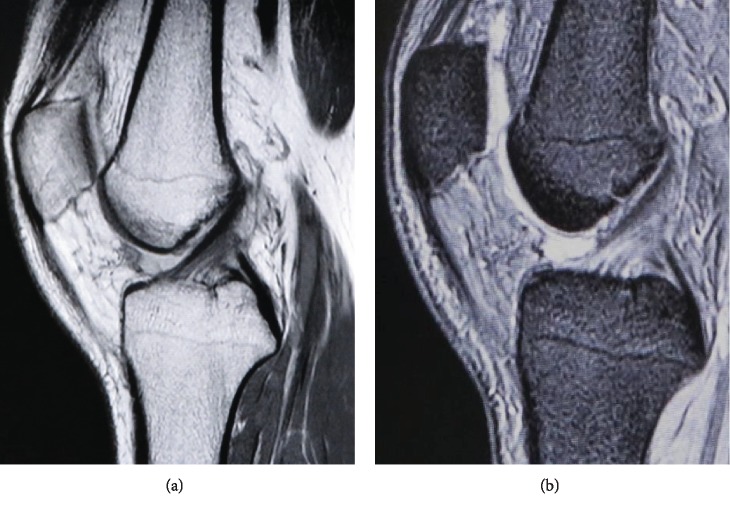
Sagittal (a) T1-weighted and (b) T2-weighted magnetic resonance images acquired one year after surgery confirm complete resolution of the intraligamentous hematoma and an intact anterior cruciate ligament.
